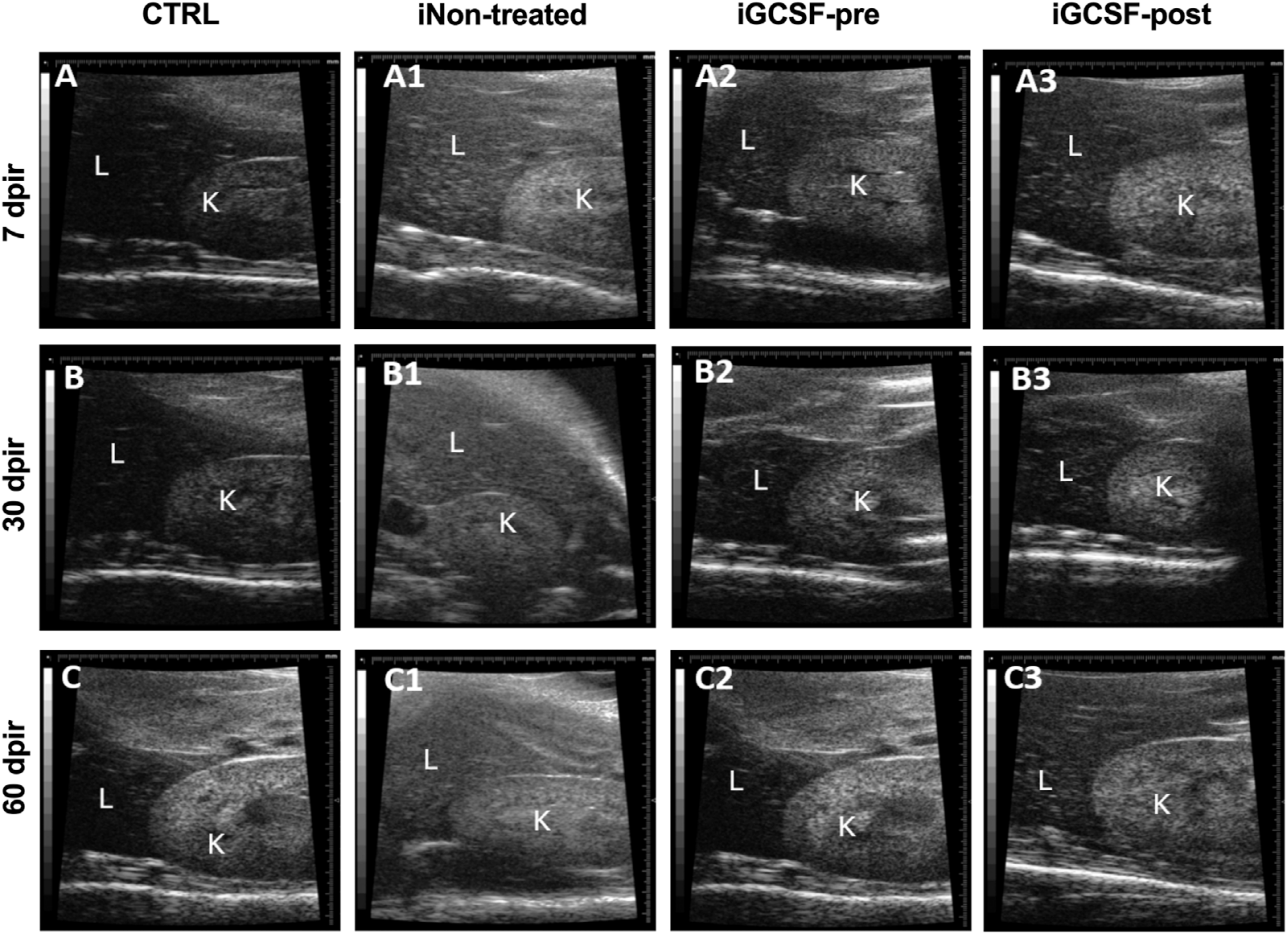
Hepatorenal ultrasound findings. Ultrasound images obtained from CTRL (A–C), iNon-treated (A1–C1), iGCSF-pre (A2–C2) and, iGCSF-post group (A3–C3) at 7, 30- and 60-day post-irradiation. Images obtained from CTRL group (A–C) and iGCSF-pre Group (A2–C2) showed that the liver (L) was less echogenic than the kidney (K). Echogenicity similarities between liver and kidney were observed in 7 dpir (A1). Changes in hepatorenal echogenicity were observed in 60 dpir in iGCSF-post, where the liver was hyperechogenic concerning the renal parenchyma (*n* = 8 per Group).
